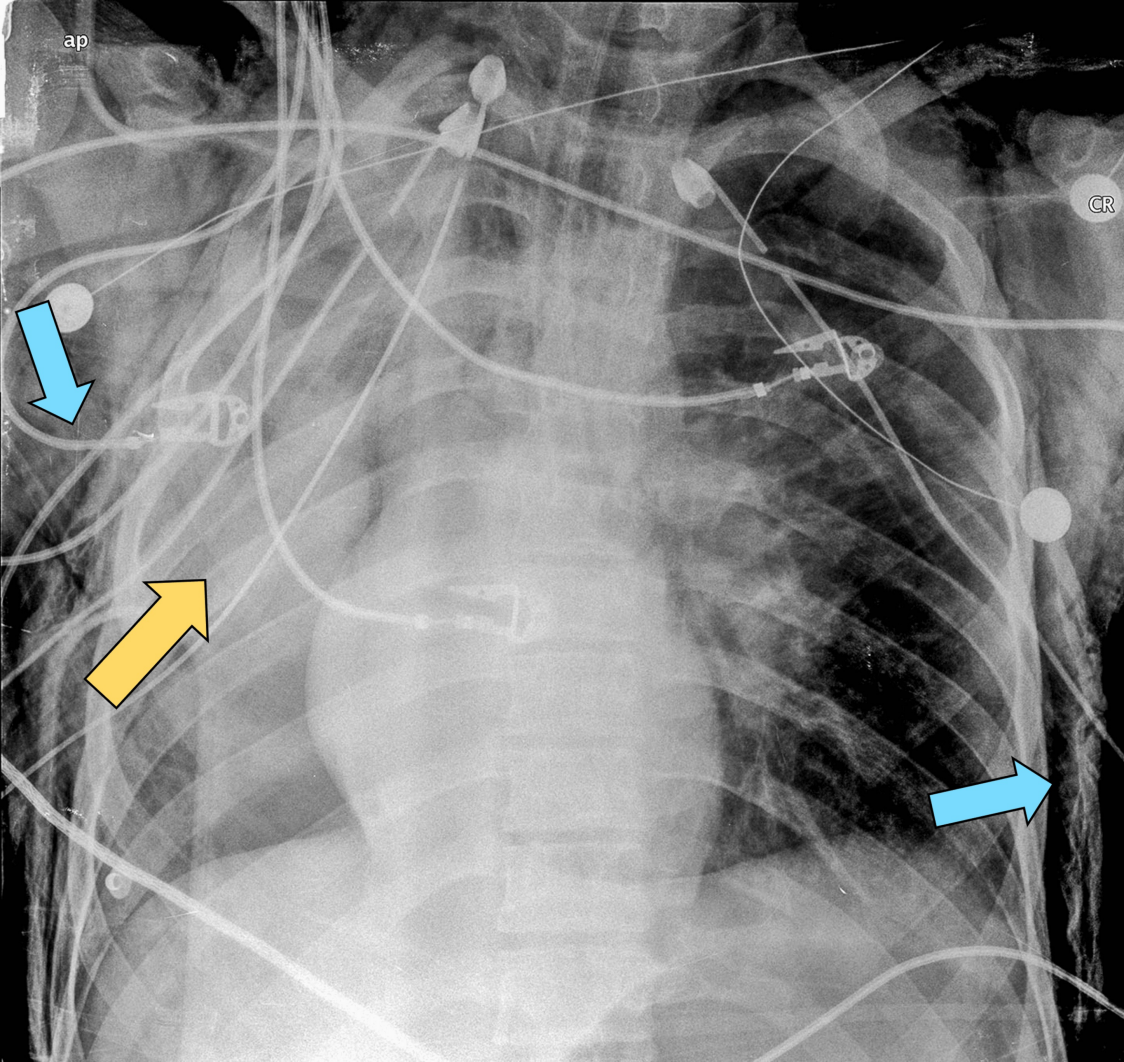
Chest X-ray showing the persistent collapse of the right lung (yellow arrow) and subcutaneous emphysema (blue arrows) despite the placement of bilateral chest tubes.
